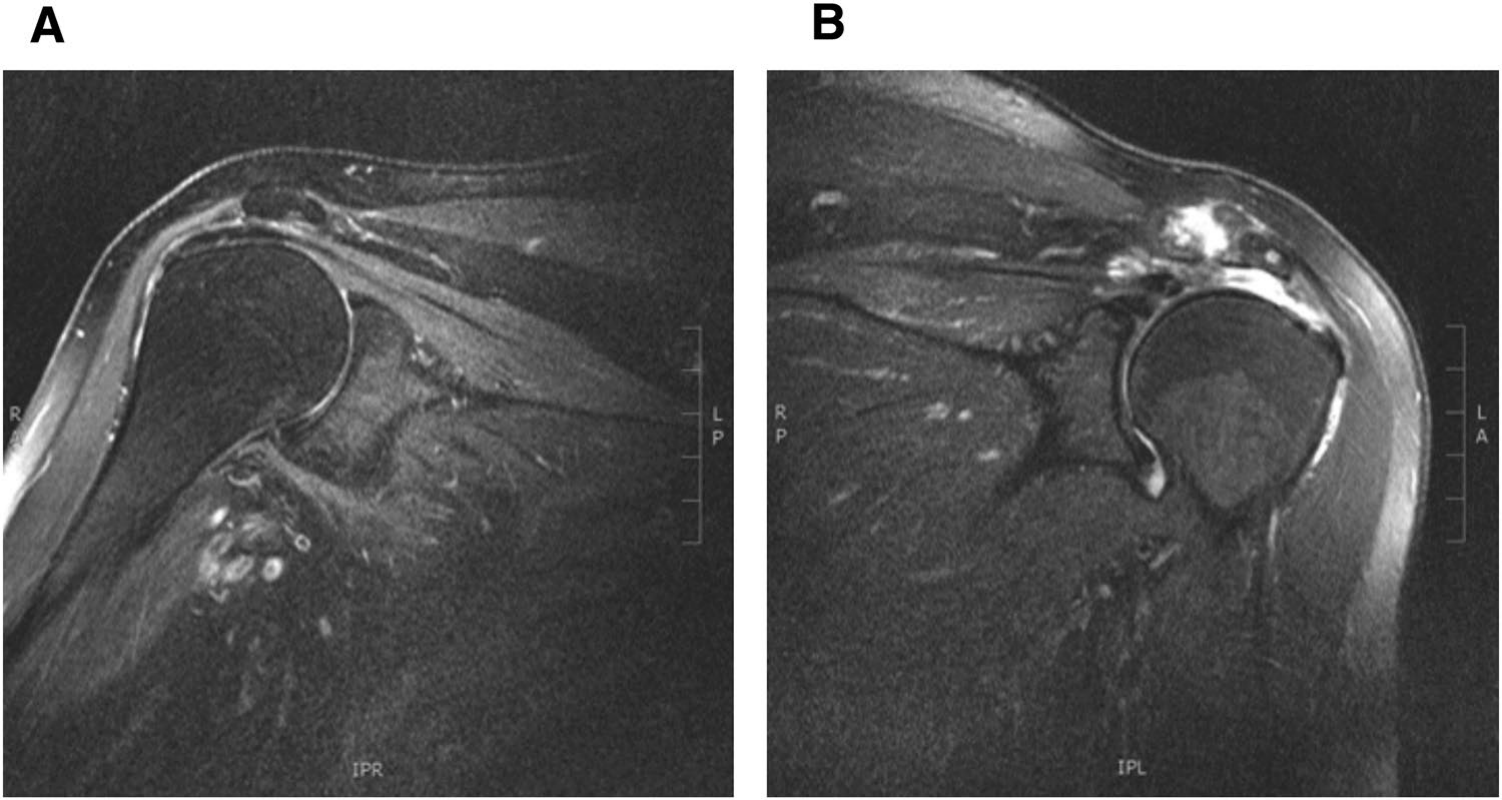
MRI-example of sagittal T2-images of a (A) right and (B) left shoulder. This patient suffered an upper limb amputation of the right side. Note the full-thickness rotator cuff tear besides other degenerative changes of the shoulder joint
